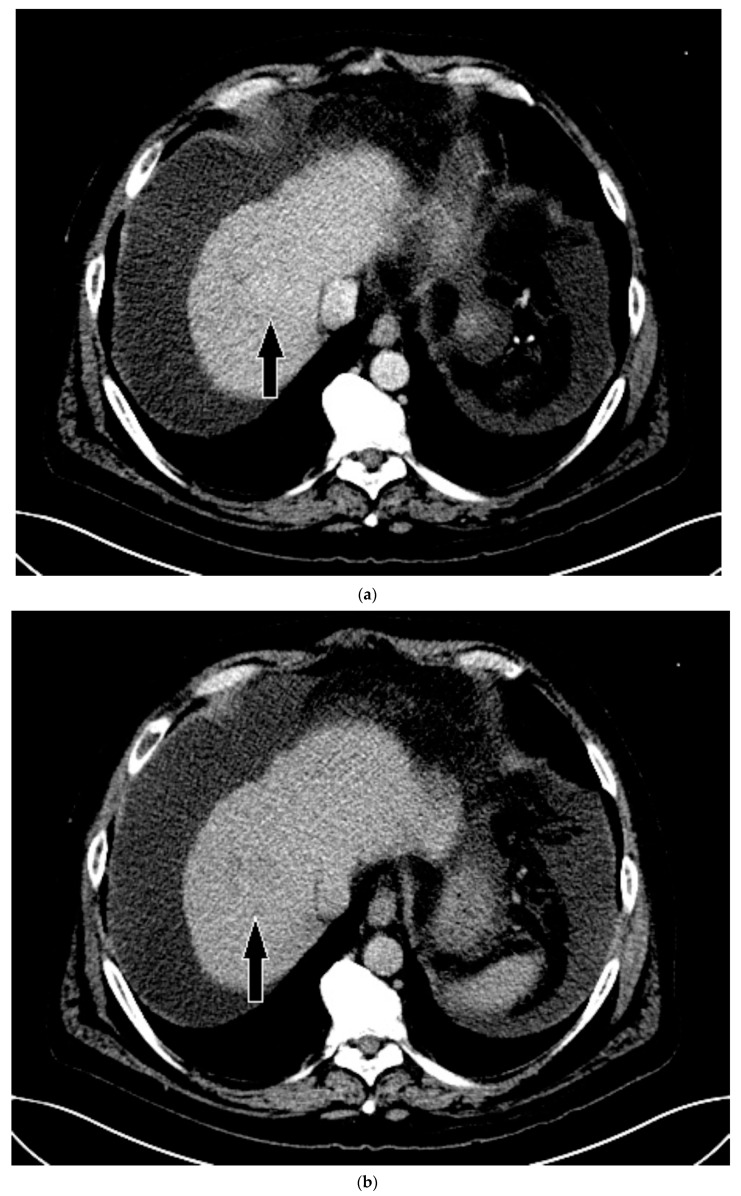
Patient 3 CT images using liver windows to highlight the subtle enhancement and washout of the lesion seen on CEUS (white arrow): (a) late arterial phase axial contrast-enhanced CT; (b) 3 min delayed phase.
